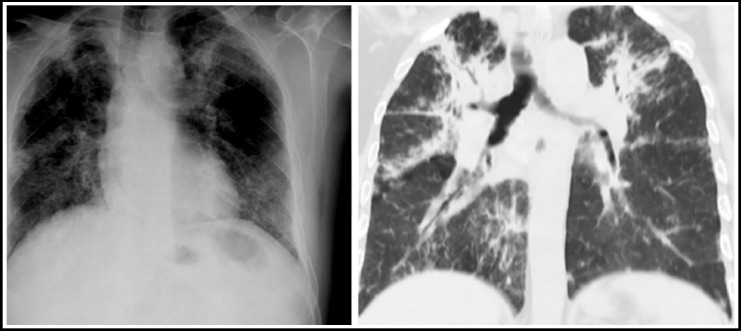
Chest X ray shows increased interstitial markings bilaterally. Corresponding HRCT shows fibrous changes in both lung fields consistent with fibrothorax.
